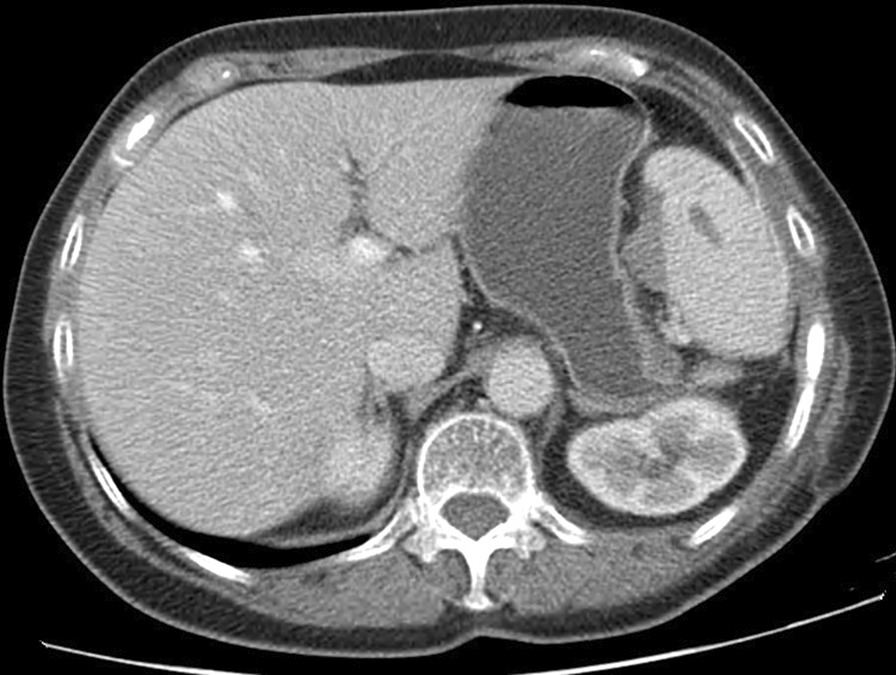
Postoperative CT, 1 month after surgery, showing tumor-free state
